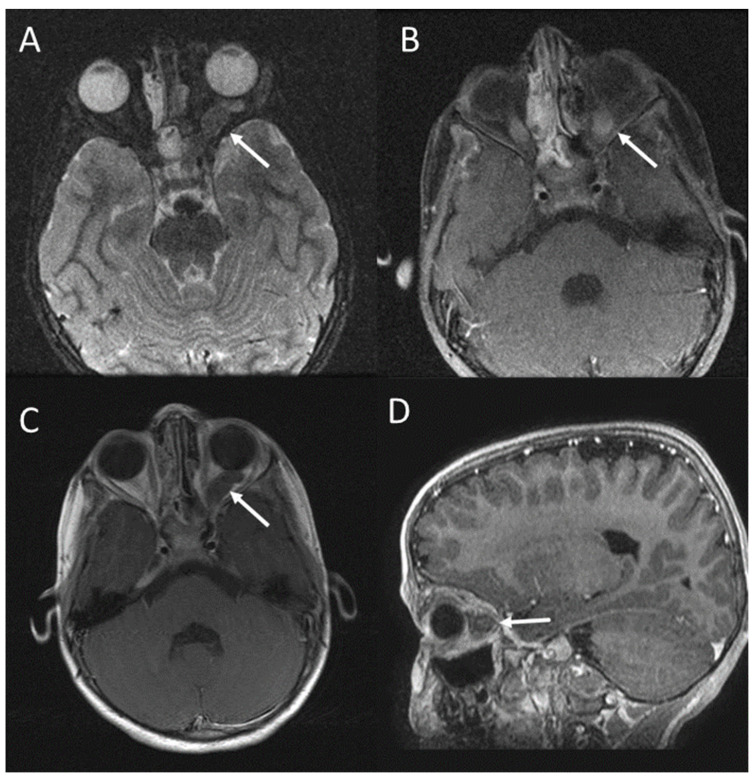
Left optic nerve glioma (arrows) in a patient with NF1. Axial T2-weighted STIR (A); axial T1-weighted after gadolinium administration with fat suppression (B); axial T1-weighted FSE after gadolinium administration (C); sagittal T1-weighted FSPGR after gadolinium administration (D) [11].
